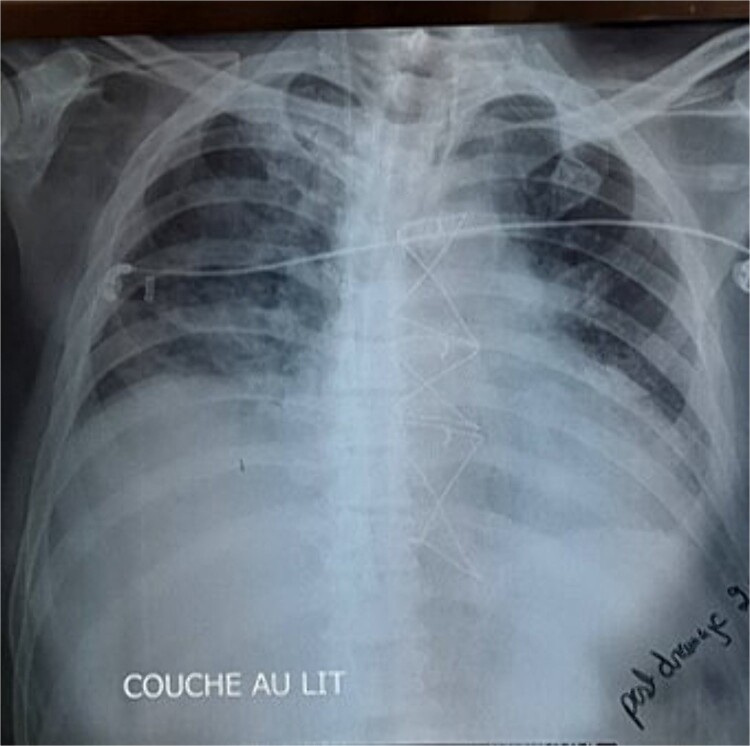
The chest X-ray showed right pulmonary edema, with total deviation of the heart to the left, as well as an elongation and straightening of the left heart border.
